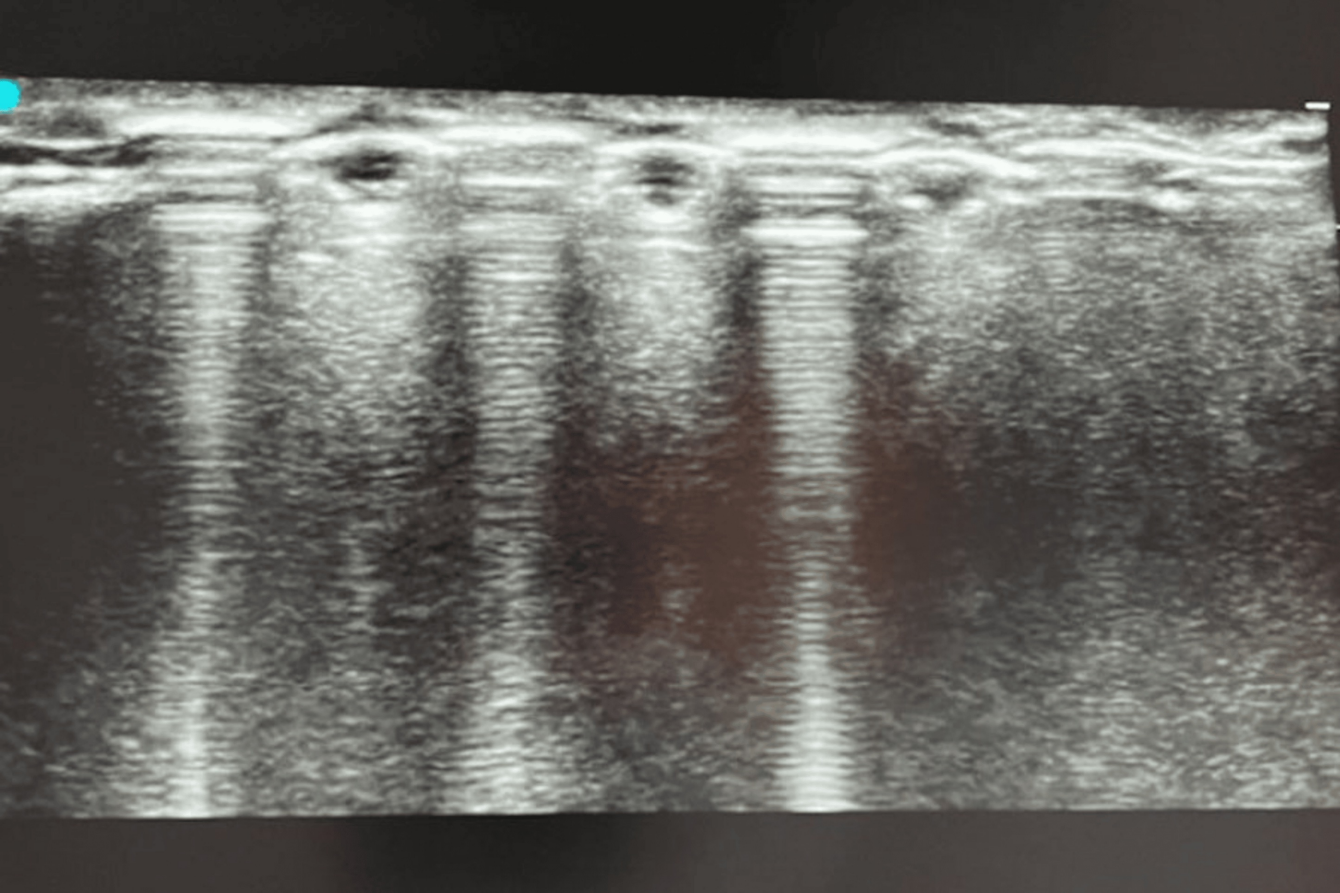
Ultrasonographic visualization of screws and plate.
